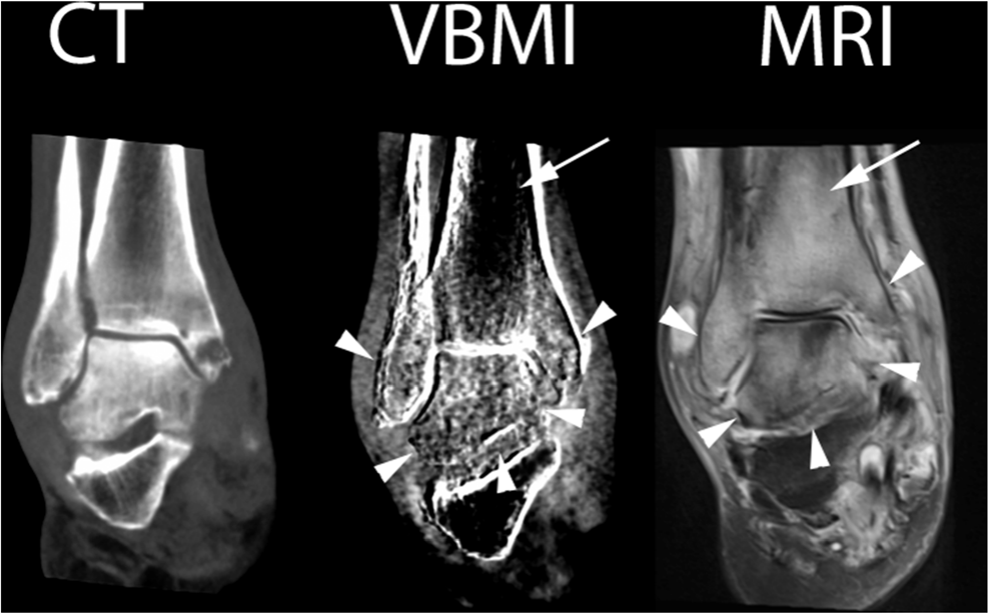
Right ankle of patient No. 11 (infectious arthritis). CT: normal CT image at 135 kV, VBMI: corresponding VBMI image, MRI: corresponding fat-saturated PD sequence – MRI shows pronounced edema in the distal tibia, fibula and calcaneus (*arrowheads*). The osteitis in the distal fibula and tibia was missed by reader 2 in VBMI. Nonetheless, VBMI depicts the edema in the distal tibia to a lesser extent compared to MRI (*arrow*)
